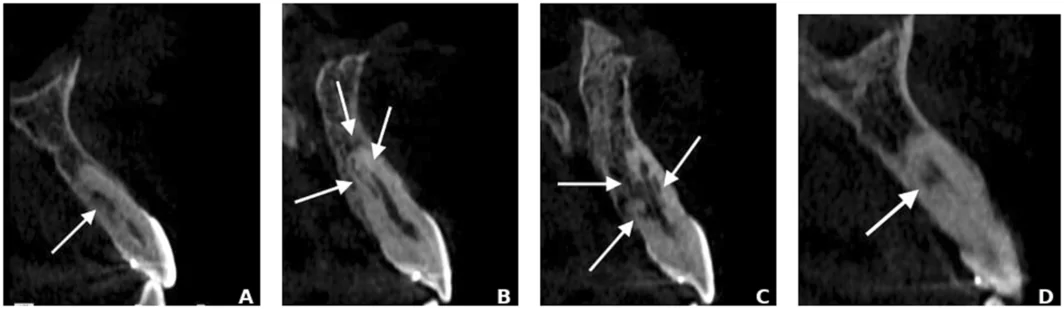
CBCT sagittal sections of the maxillary incisors, obtained on December 11, 2017. (A) Sagittal section of a maxillary incisor. (B) Sagittal section of a maxillary incisor, with arrows indicating the area of resorption. (C) Sagittal section of a maxillary incisor, with arrows indicating the area of resorption. (D) Sagittal section of a maxillary incisor, with arrows indicating the area of resorption.
